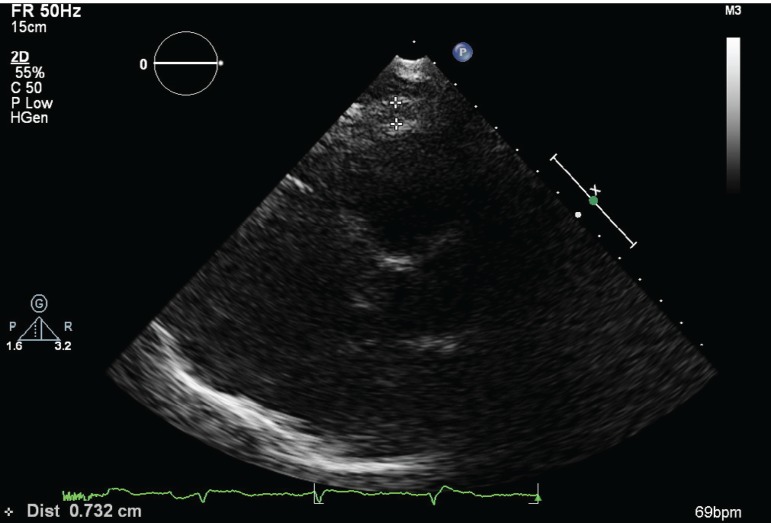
Measurement of epicardial fat thickness by echocardiography.
